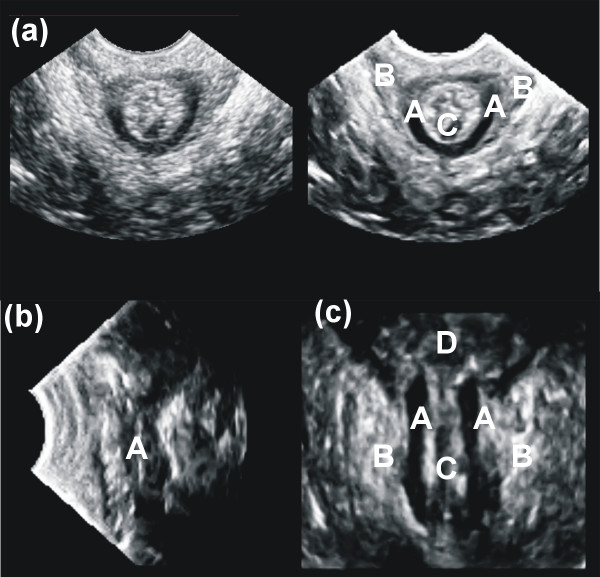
Sonographical data. Sonographical documentation method for examination of the pelvic floor in analogon to the usual used MR nomenclature [36, 37]. Shown is the filtered US data set: (a) axial, (left side: not filtered to show the enhancement induced by filtering) (b) sagittal and (c) coronal plane through the anal canal. A: the internal anal sphincter muscle; B: the external anal sphincter muscle (levator ani muscle); C: anal canal mucosa; D: rectum.
